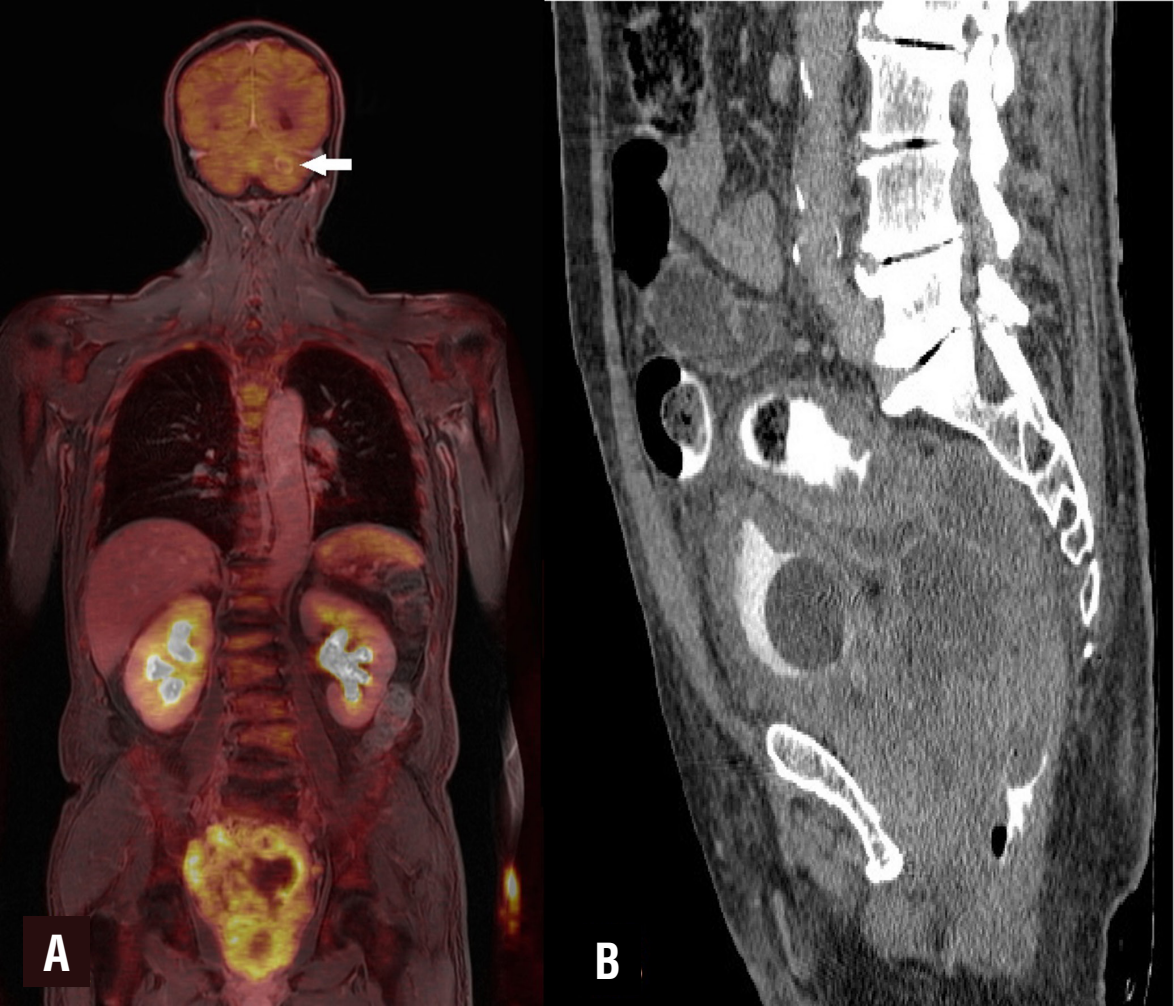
A) Coronal view of the tumor with PET-MR. (White arrow shows cerebellar metastases), B) Sagittal reconstruction of CT images showing tumor compression to the rectum.
